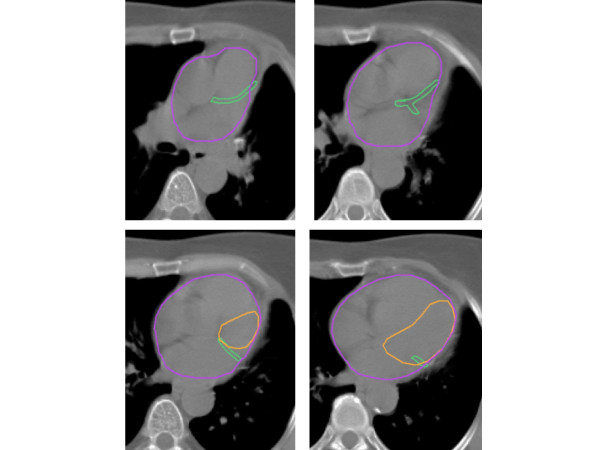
Treatment planning computed tomography scan with contoured left anterior descending coronary artery (and part of the left circumflex artery) in green color, left ventricle in orange and heart in purple.
